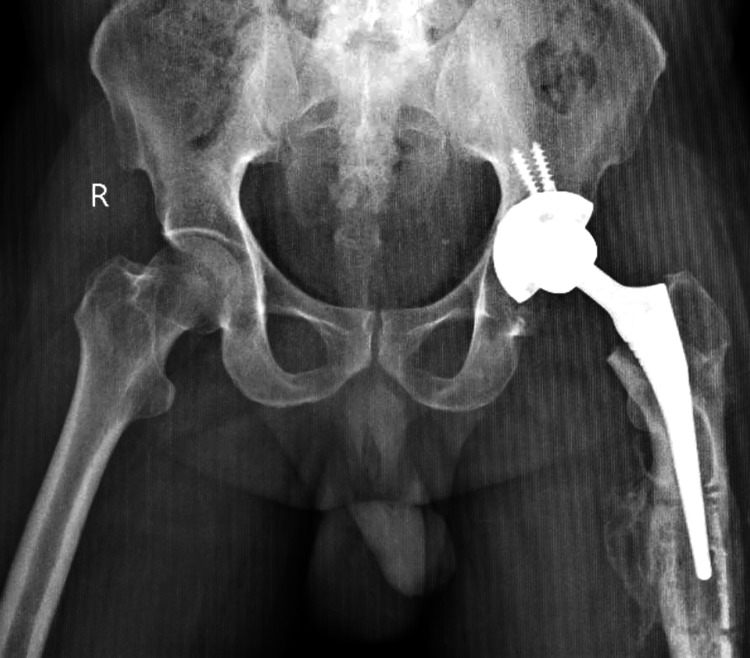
X-ray of the operated case of total hip replacement on the left side with the implant in situ.
